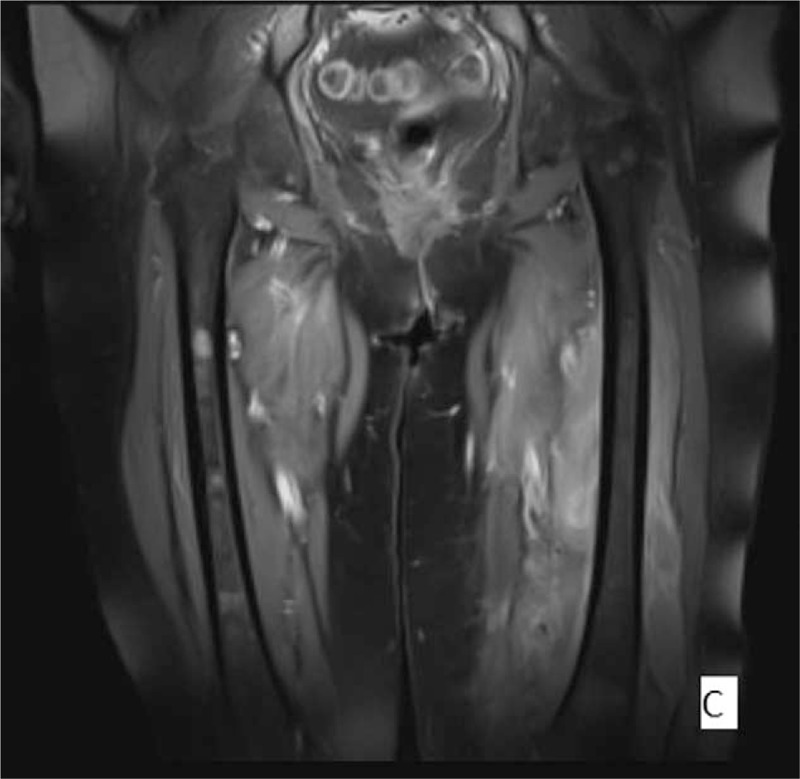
Left- suppressed contrast enhanced T1-weighted image (Image 3) and Image 2 show multiple foci of T1 hypointense and enhancing marrow lesions in both femora and extensive multicompartmental soft tissue mass involvement with peripheral thick band-like enhancement.^[4]^
